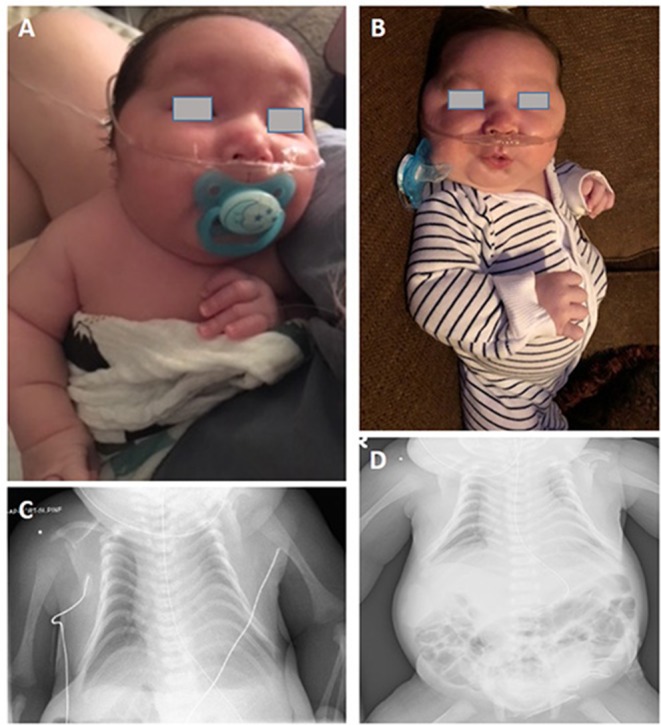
(A,B) Photograph of the patient. (C) Chest radiography of the patient. Thoracic dysplasia (bell-shaped small thorax with coat-hanger appearance). (D) Anterior abdominal wall defect with bowel herniation consistent with history of omphalocele. (The written informed consent was obtained from the parents of the patient for the publication of this image).
